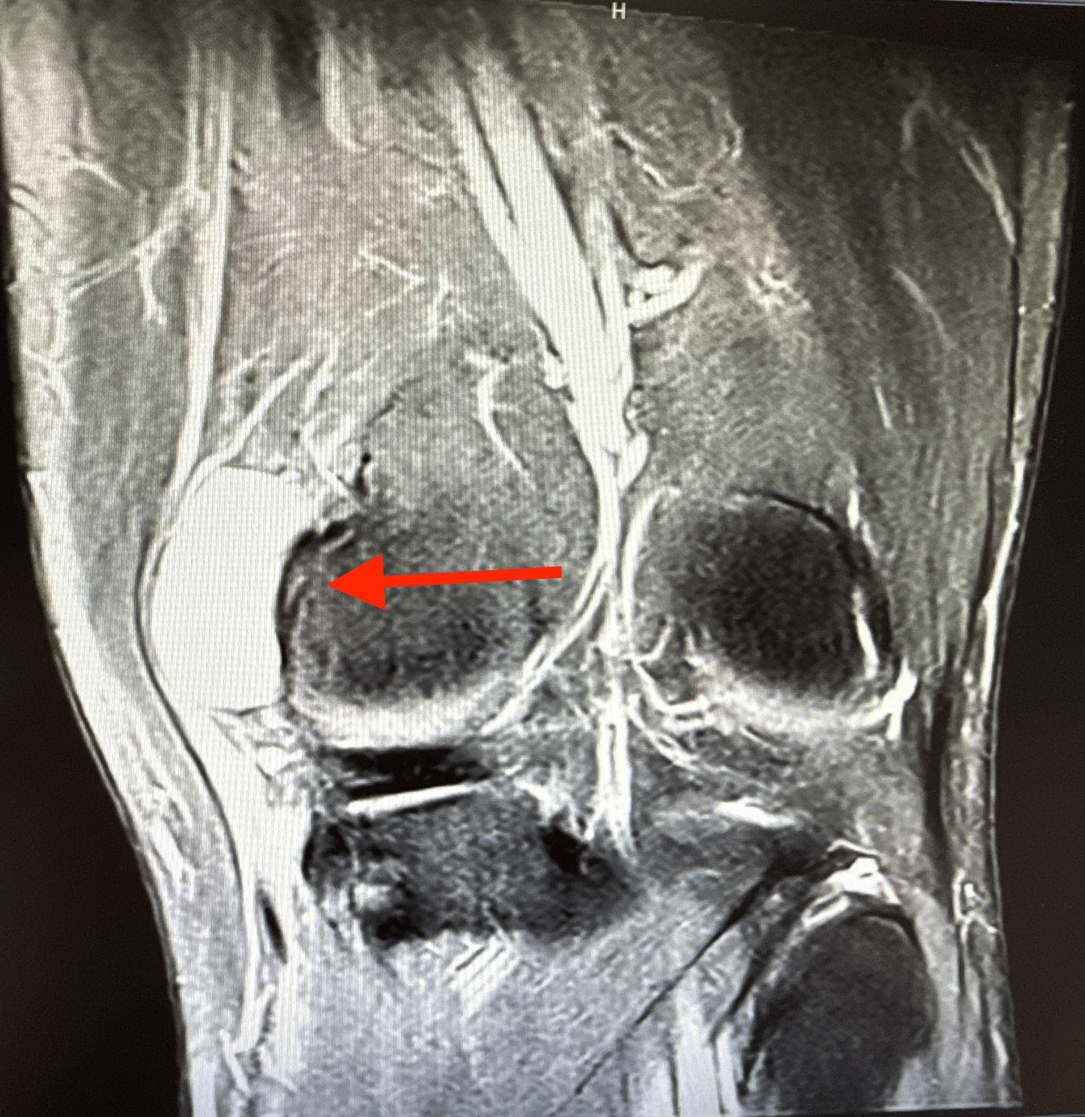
Coronal MRI of the knee with hyperdensity, which suggests fluid collection (arrow).
